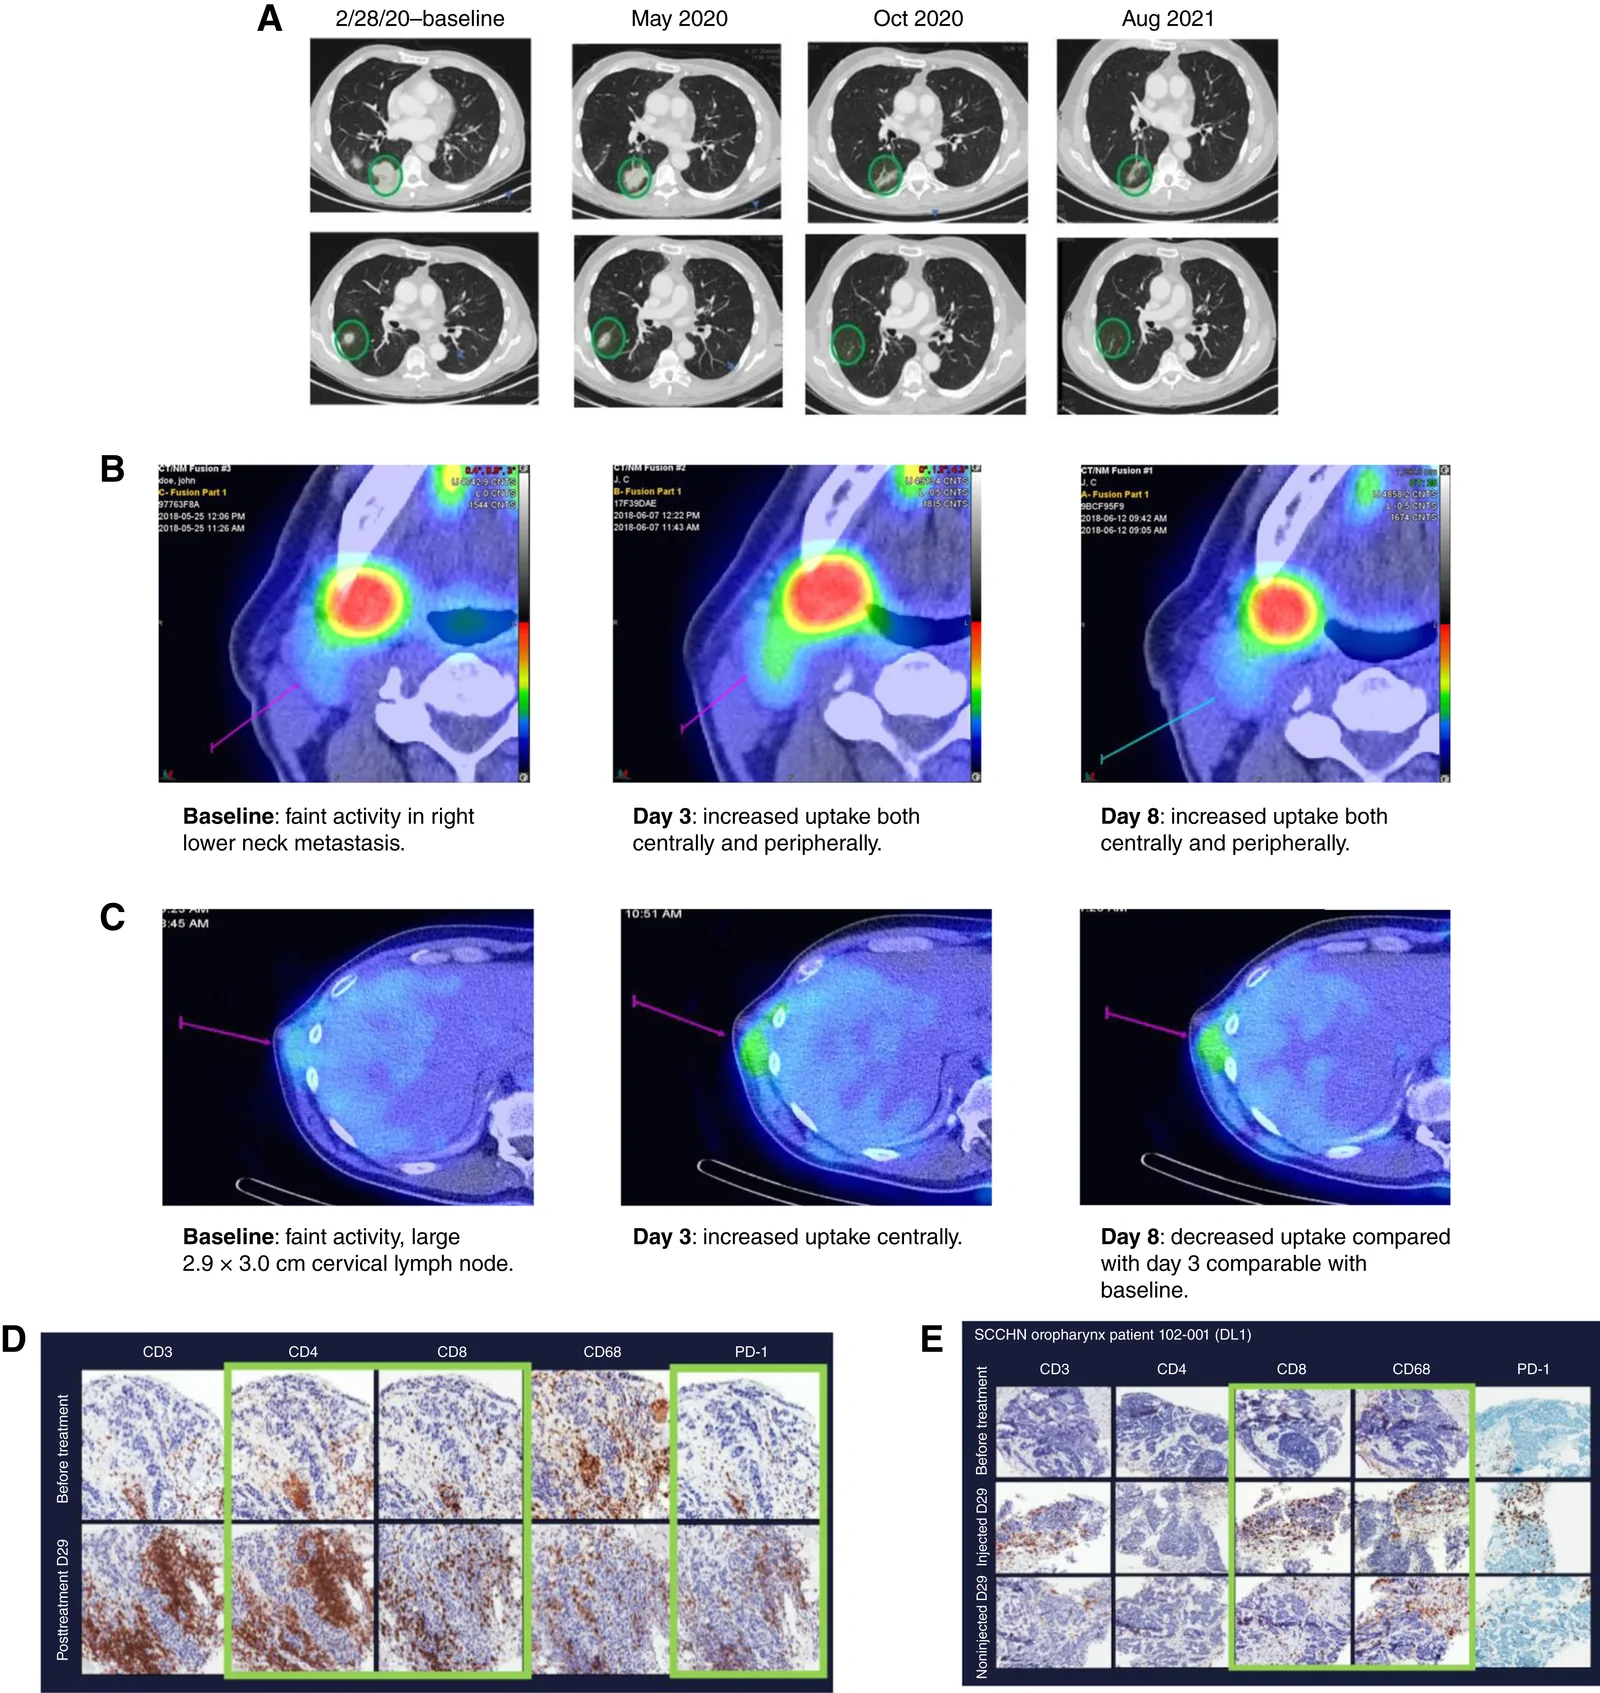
Imaging following intratumoral (IT) and intravenous (IV) VV1. A, CT images of the thoracic region of a 57-year-old patient with thymic carcinoma following a 30-minute intravenous infusion of VV1 (1.7 × 10^10^ TCID_50_) + labeled dose/regimen of avelumab (part 3). In the opinion of the investigator, only scar tissue remained at the last assessment (August 2021). B, SPECT/CT/NIS imaging of 75-year-old male patient with adenocarcinoma (unknown primary cancer) following intratumoral (IT) administration of VV1 (3 × 10^8^ TCID_50_) monotherapy (part 1). C, SPECT/CT/NIS imaging of a 62-year-old male patient with mesothelioma following IT administration of VV1 (1 × 10^8^ TCID_50_) monotherapy (part 1). D, Immune cell infiltrates and PD-1 in a male patient with head and neck squamous cell (HNSCC) with SD, following IT administration of VV1 (part 1). Images are of an injected lesion. E, Immune cell infiltrates and PD-1 in a 70-year-old male patient with HNSCC with SD, following IT administration of VV1 (3 × 10^6^ TCID_50_) monotherapy (part 1). Top, baseline. Middle, injected lesion. Bottom, noninjected lesion.
